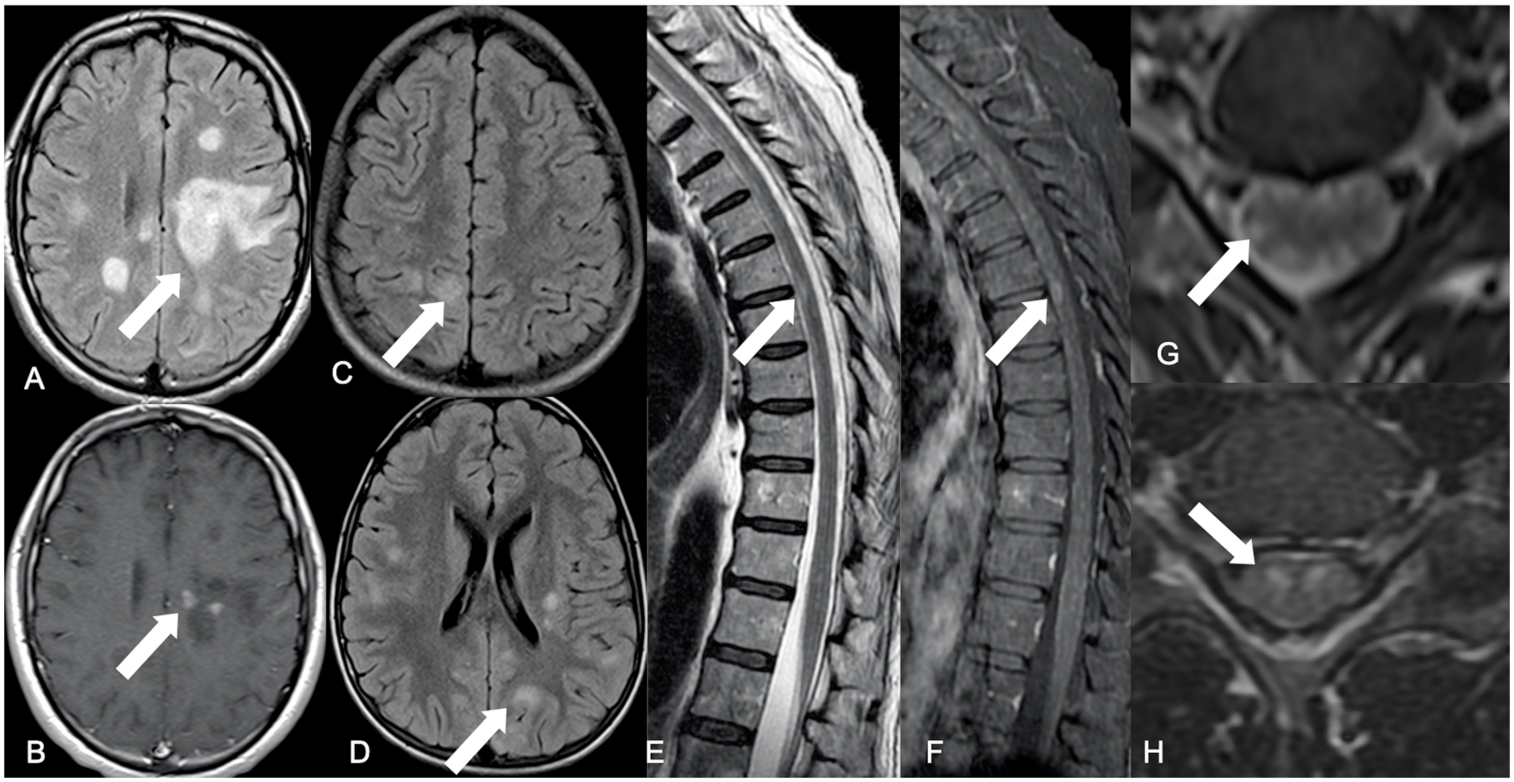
Summary of main neuroimaging findings. Brain axial FLAIR showing a tumefactive hyperintense lesion located in the frontal-parietal transition of the left hemisphere (white arrow) (A), with punctate discontinued contrast enhancement on the corresponding axial post-contrast T1-WI (white arrow) (B), representing an ADEM pattern. An encephalitis pattern can be seen on brain FLAIR axial images (white arrows) with cortical-subcortical hyperintense diffuse lesions (C,D). Dorsal spinal cord sagittal T2-WI with elongated areas of peripherally located hypersignal intensity among the white matter tracts (white arrow) (E), associated with contrast enhancement on sagittal FS T1-WI (white arrow) (F). Axial T2-WI have elongated hypersignal images in a radial distribution, from the central spinal cord gray matter to the peripheral white matter (white arrow) (G). Axial T2-WI with hypersignal affecting the gray matter anterior horn, in a polio-like pattern (white arrow) (H).
